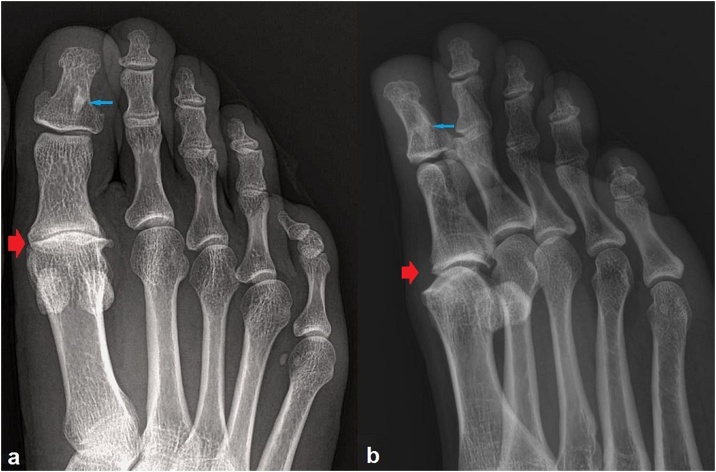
Anteroposterior and lateral-oblique foot radiographs demonstrating an osteoid osteoma in the distal phalanx (blue arrows) and degenerative disease involving the interphalangeal joint (red arrows) of the hallux.
